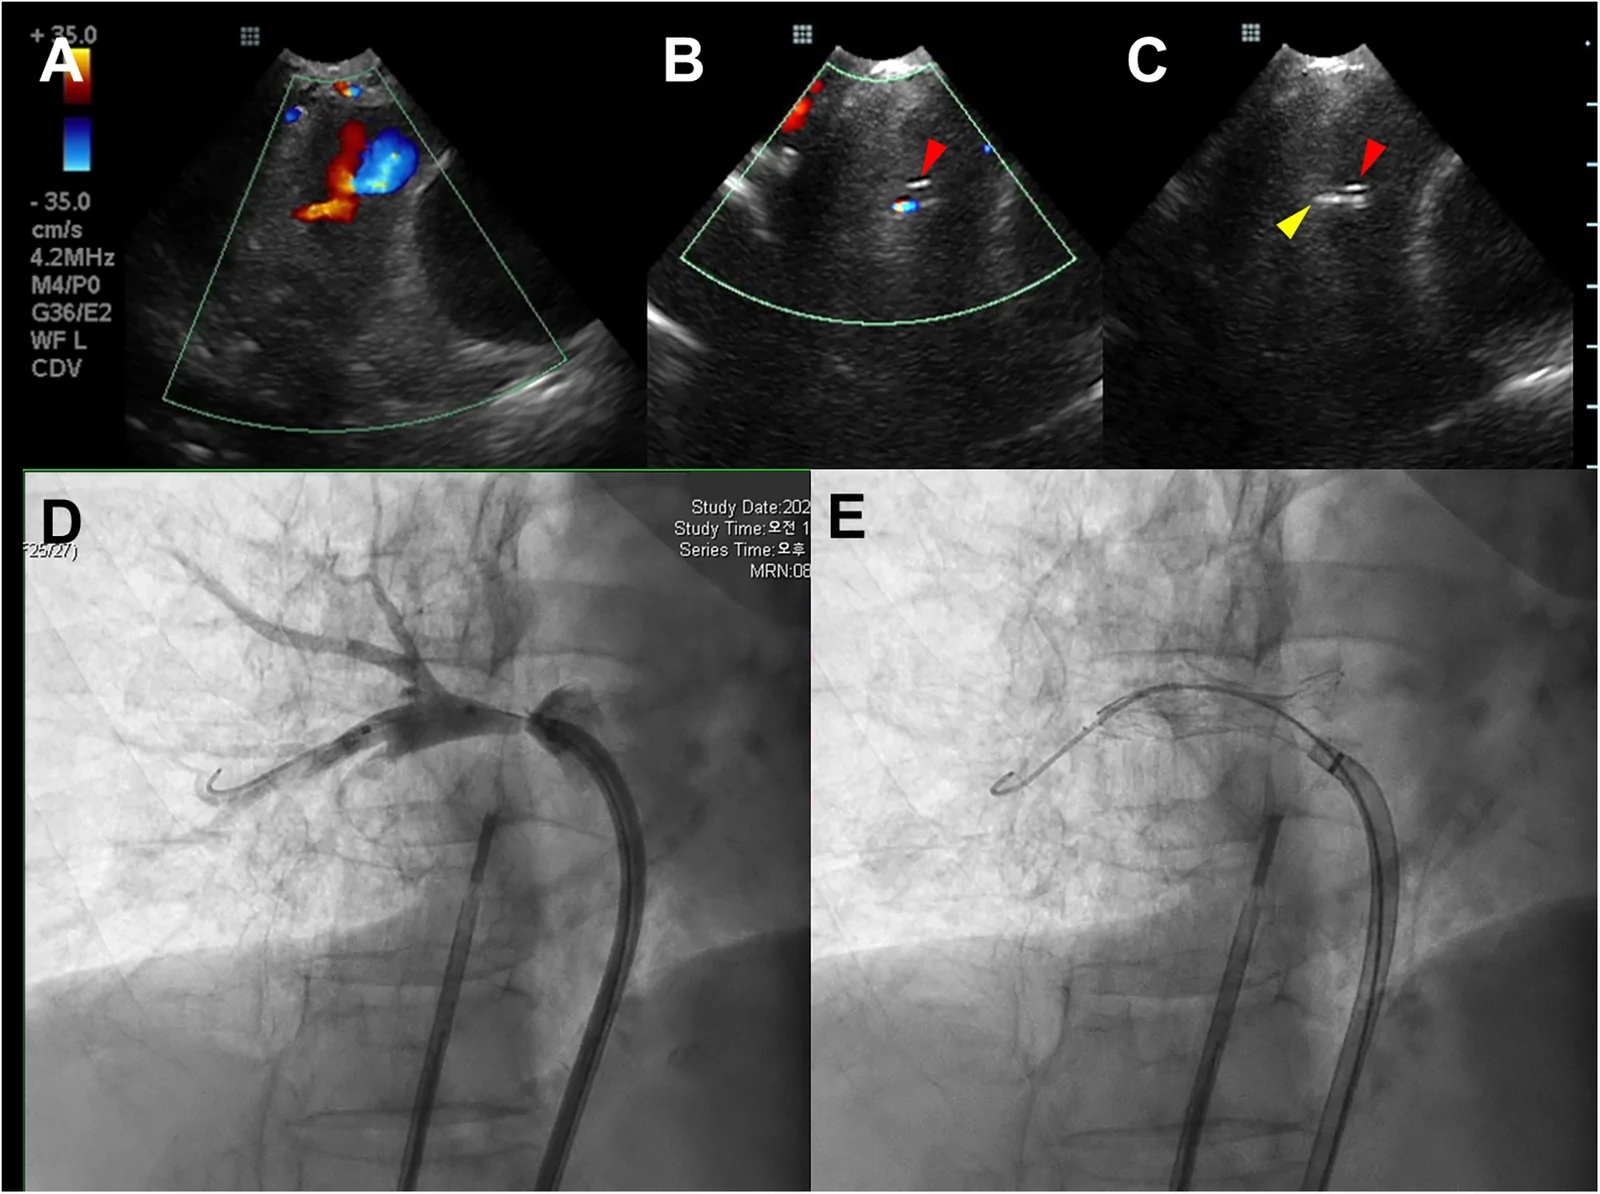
Intracardiac echocardiography guided wiring and stenting in right inferior pulmonary vein. (A) Intracardiac echocardiography showing inflow jet from the right inferior pulmonary vein. (B) The guiding sheath is deflected tracking the small inflow jet color. The sheath tip (red arrow head) and inflow jet are visible. (C) A Radifocus® guidewire (Terumo, Tokyo, Japan) (yellow arrow head) is engaged into the right inferior pulmonary vein ostium through the sheath (red arrow head). (D) and (E) Angiogram showed critical stenosis at the right inferior pulmonary vein ostium. After balloon angioplasty, a 14 × 40 mm Protégé™ GPS self-expanding peripheral stent was implanted with post adjunctive balloon dilatation.
